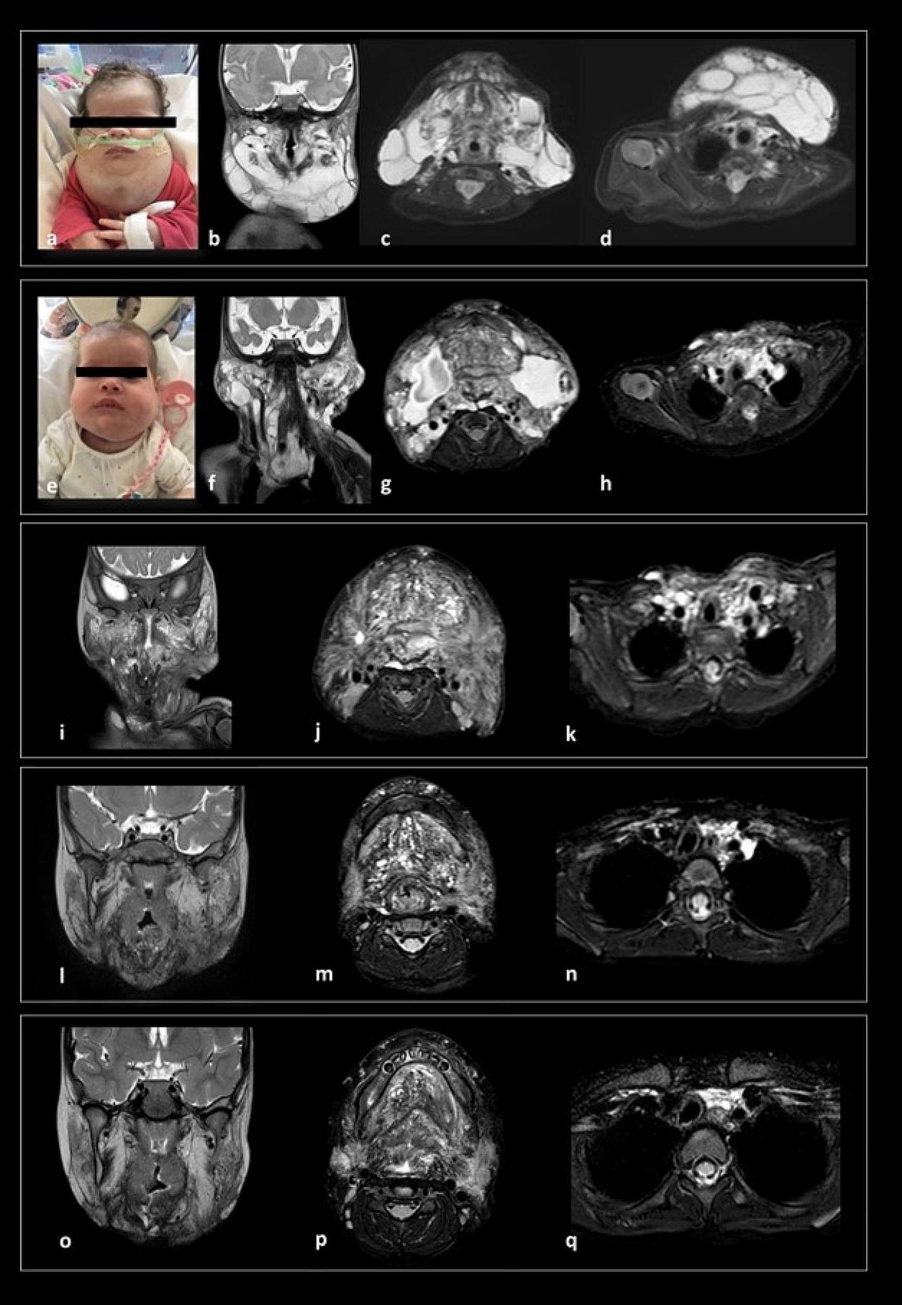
(a) Picture at birth showing a large soft swelling involving the neck, with tongue hypertrophy. MRI after birth (b, c,d) showed a voluminous macro- and microcystic LM involving the tongue, and with bilateral extensive involvement of the suprahyoid spaces (sublingual, submandibular and parotid spaces) and caudal spread up to the upper mediastinum, along with the parapharyngeal, retropharyngeal, vascular and visceral neck spaces. Persistent swelling of the neck was evident soon after surgery and sclerotherapy (e). MRI study (f, g, h) performed 10 days after these procedures documented a mild enlargement of the LM in the deep spaces of the neck and in the upper mediastinum, with microcystic involvement of the tongue and oropharyngeal walls causing pharyngeal air column obstruction needing tracheostomy placement. An increased volume of superficial macrocystic components was also detected. The serial follow-up MRI scans during sirolimus therapy (7 months 22 day – i, j, k, 2 year 8 months – l, m, n, 6 years – o, p, q) show progressive volumetric reduction of the LMs, especially the upper mediastinal components (left column, coronal view, T2-w; middle and right column, axial view, T2-w fat sat) respectively passing through the oropharynx and the upper mediastinum. The last US examination (j) shows absence of residual macrocystic components in the superficial neck spaces
